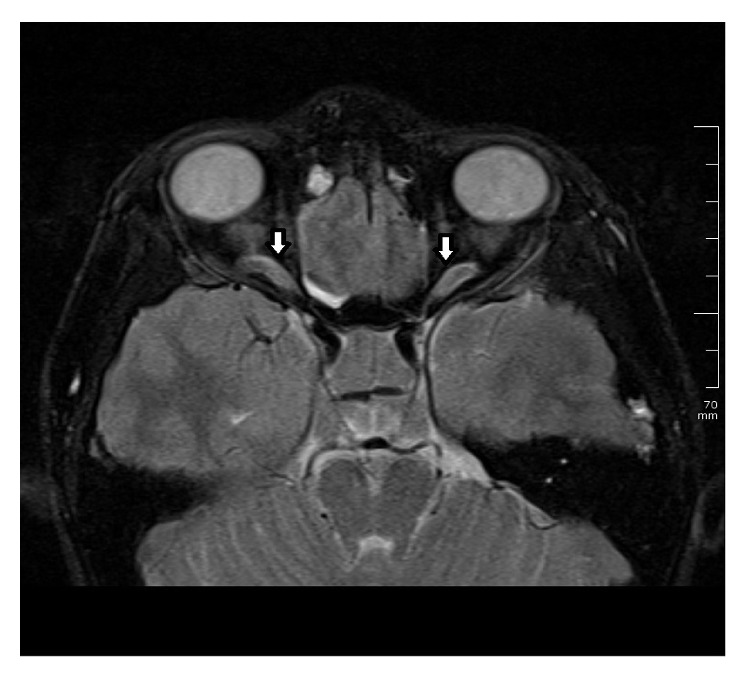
Magnetic resonance imaging. Contrast-enhanced axial T2-weighted MRI scan shows cerebrospinal fluid tracking along the optic nerve sheath.
